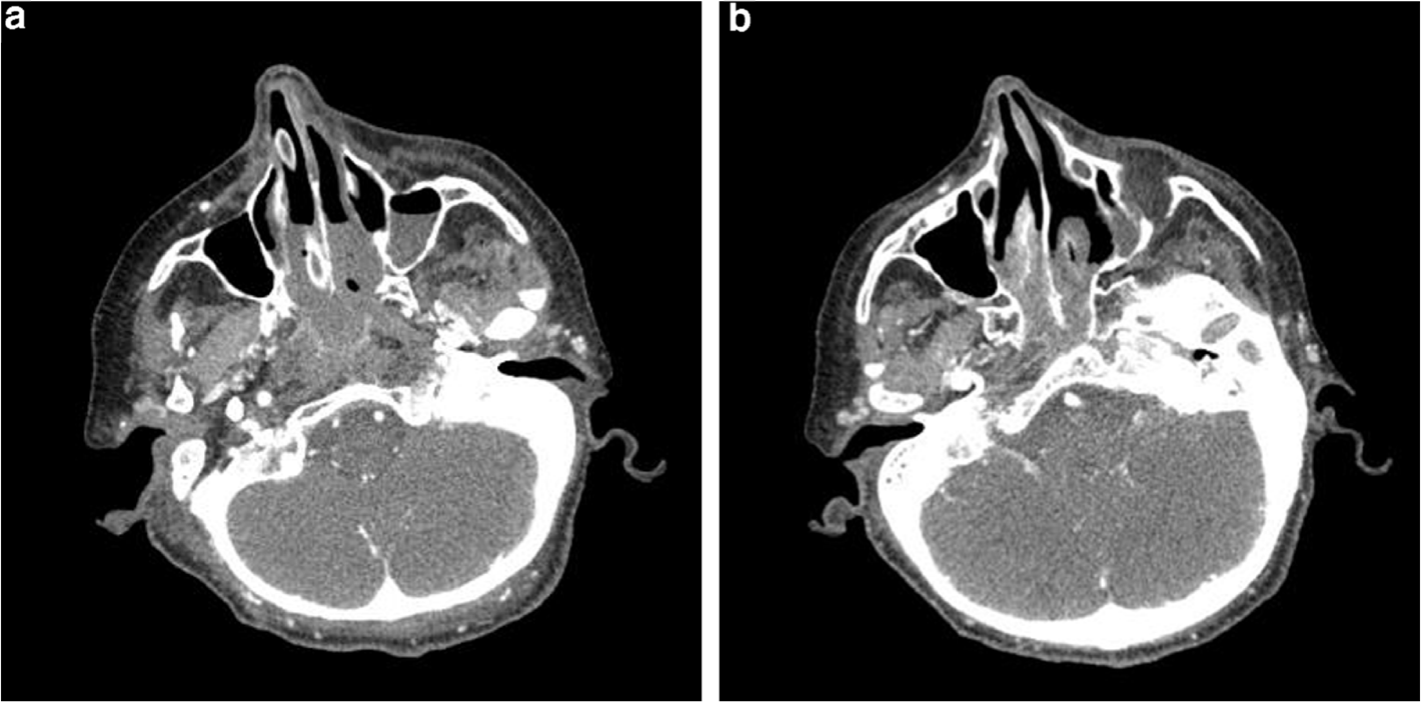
(a and b) A brain computed tomography scan showing blurred bony structures of the floor of the mouth and the tongue, consolidation of left maxillary sinus and accompanying invasion of the maxillary bone.
